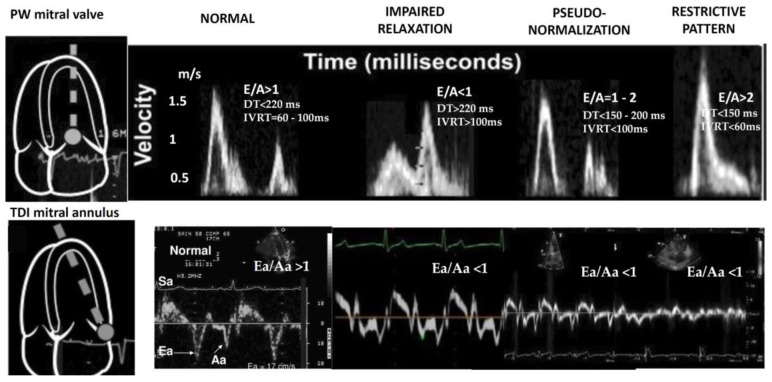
Diastolic filling patterns evaluated by pulsed-wave Doppler and TDI.
